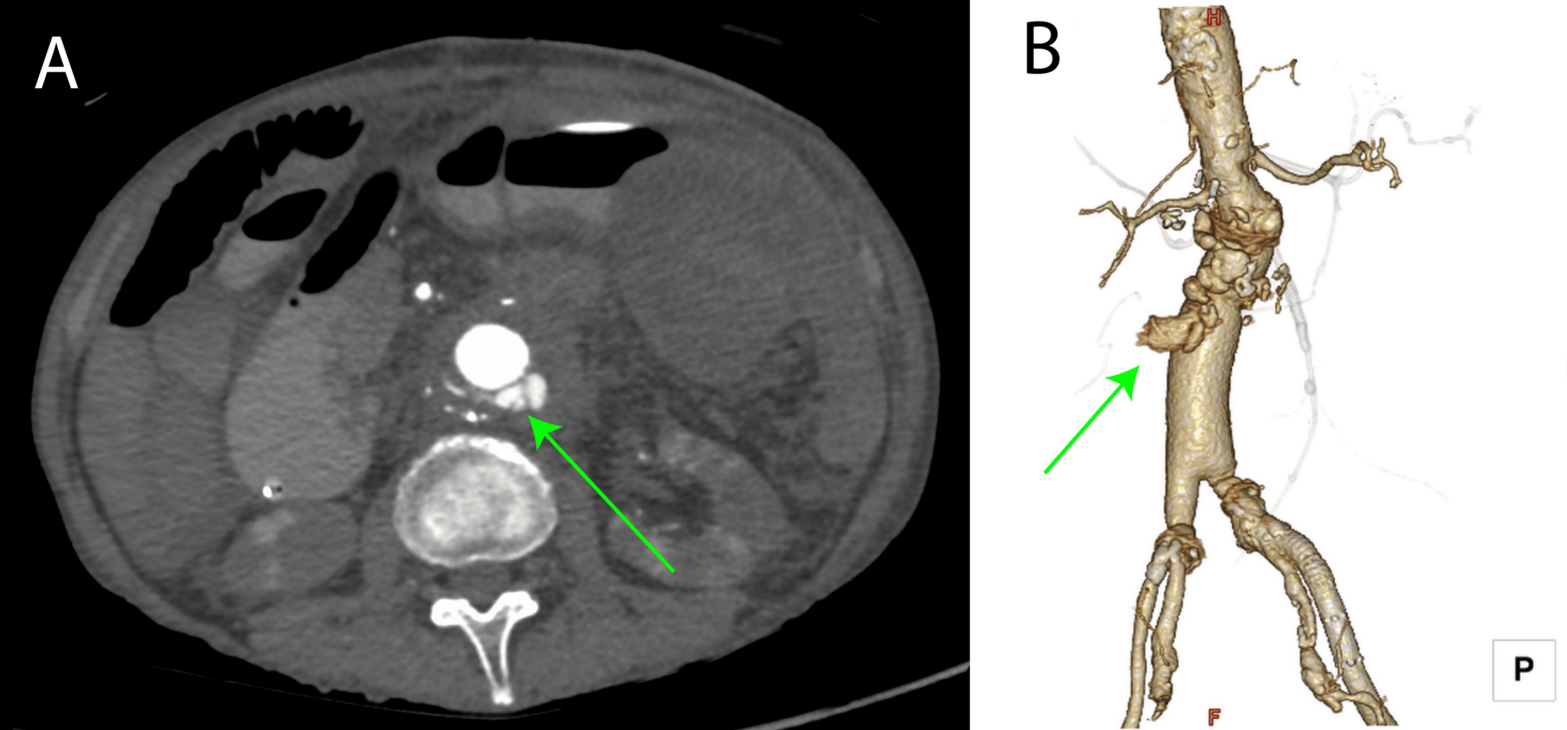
Postoperative enhanced computed tomography (A) Horizontal section image. Bleeding from the central artificial vascular anastomosis was observed, bleeding dorsally. (green arrow); (B) Volume rendering image. The dorsal view showed hemorrhage. (green arrow).
